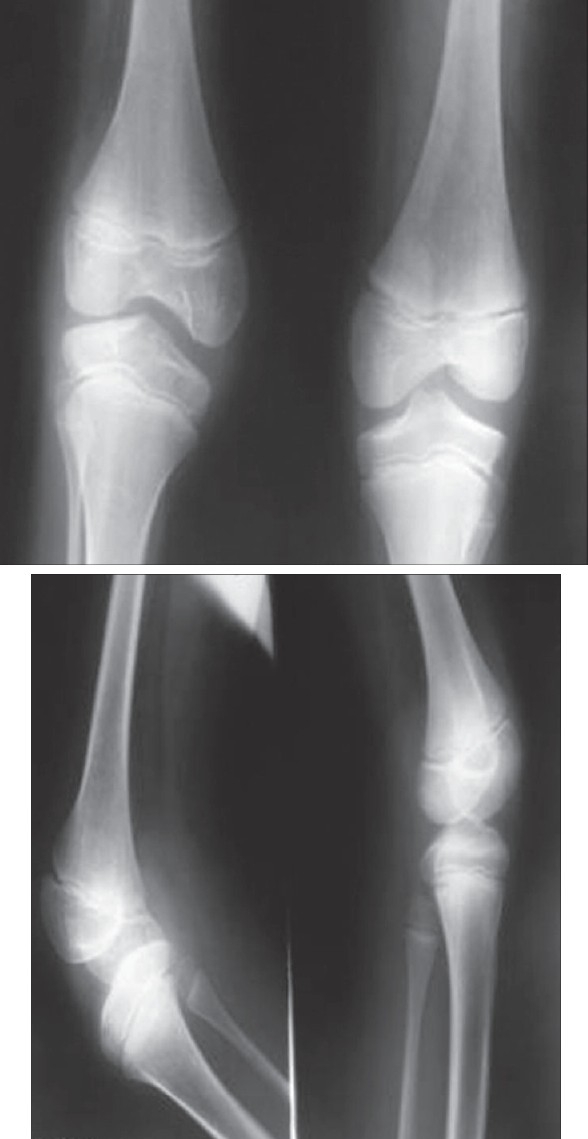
Radiograph shows the absence of both patellae and large femoral condyles in antero-posterior and lateral views
